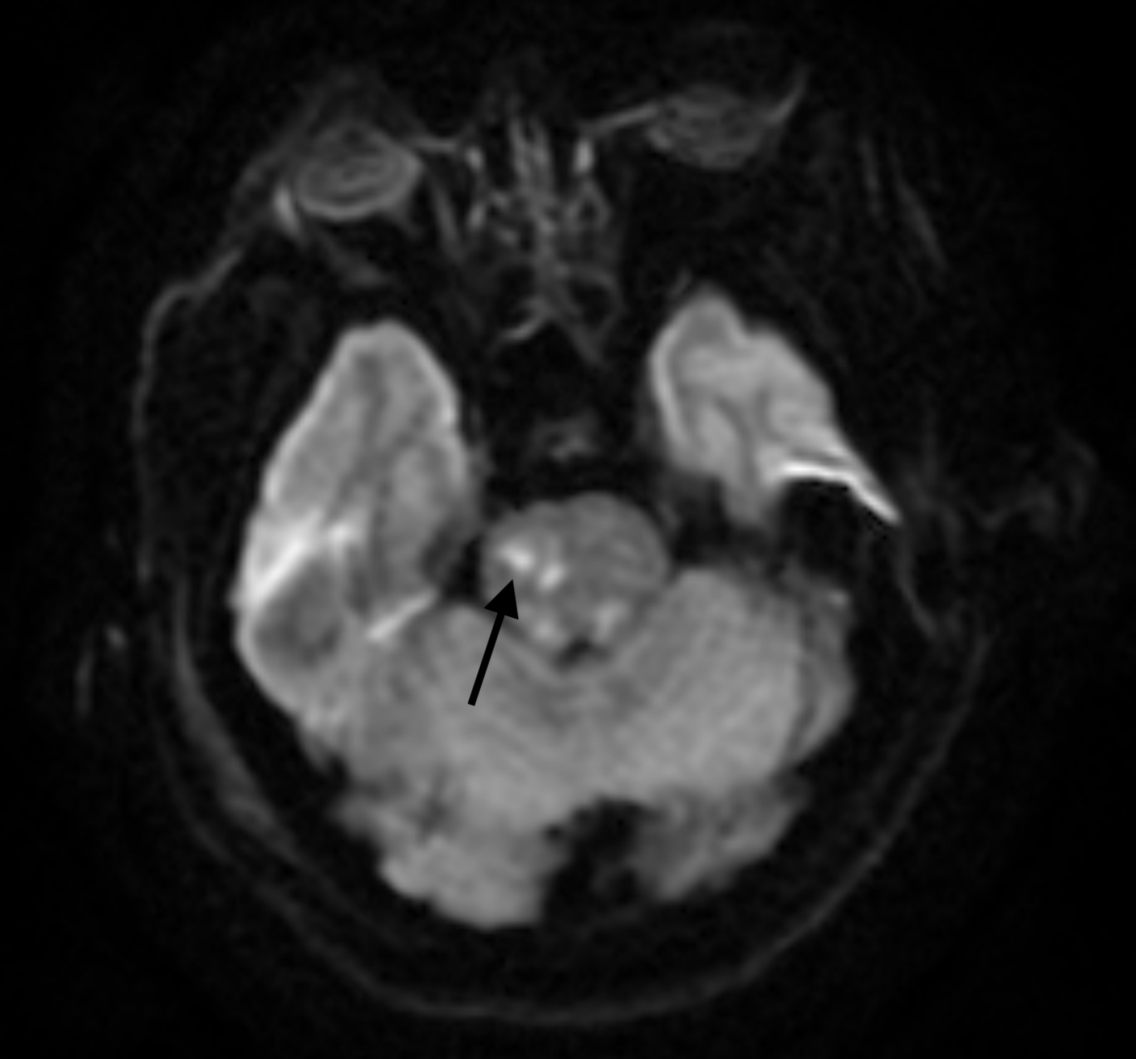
MRI brain after drain removal MRI of the brain without gadolinium. Imaging completed after removal of both subdural drains. There is evidence of two, new, right-sided, punctate, pontine ischemic strokes.
